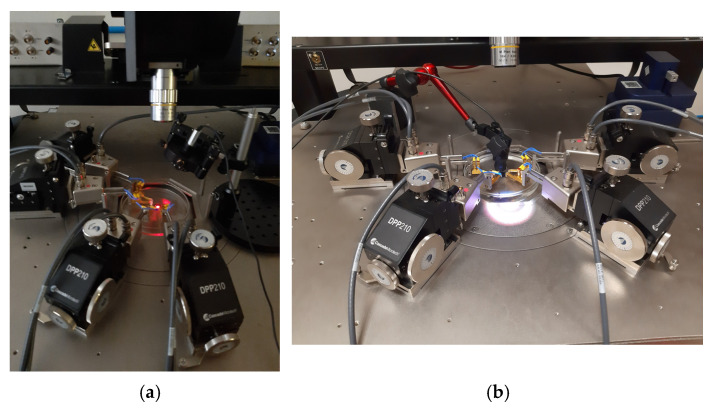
Experimental setup showing close up views of the probe station with close up views of (a) The laser diffraction experiment and (b) the acoustic testing setup showing the red supporting structure for the hydrophone/ultrasonic projector [7].
